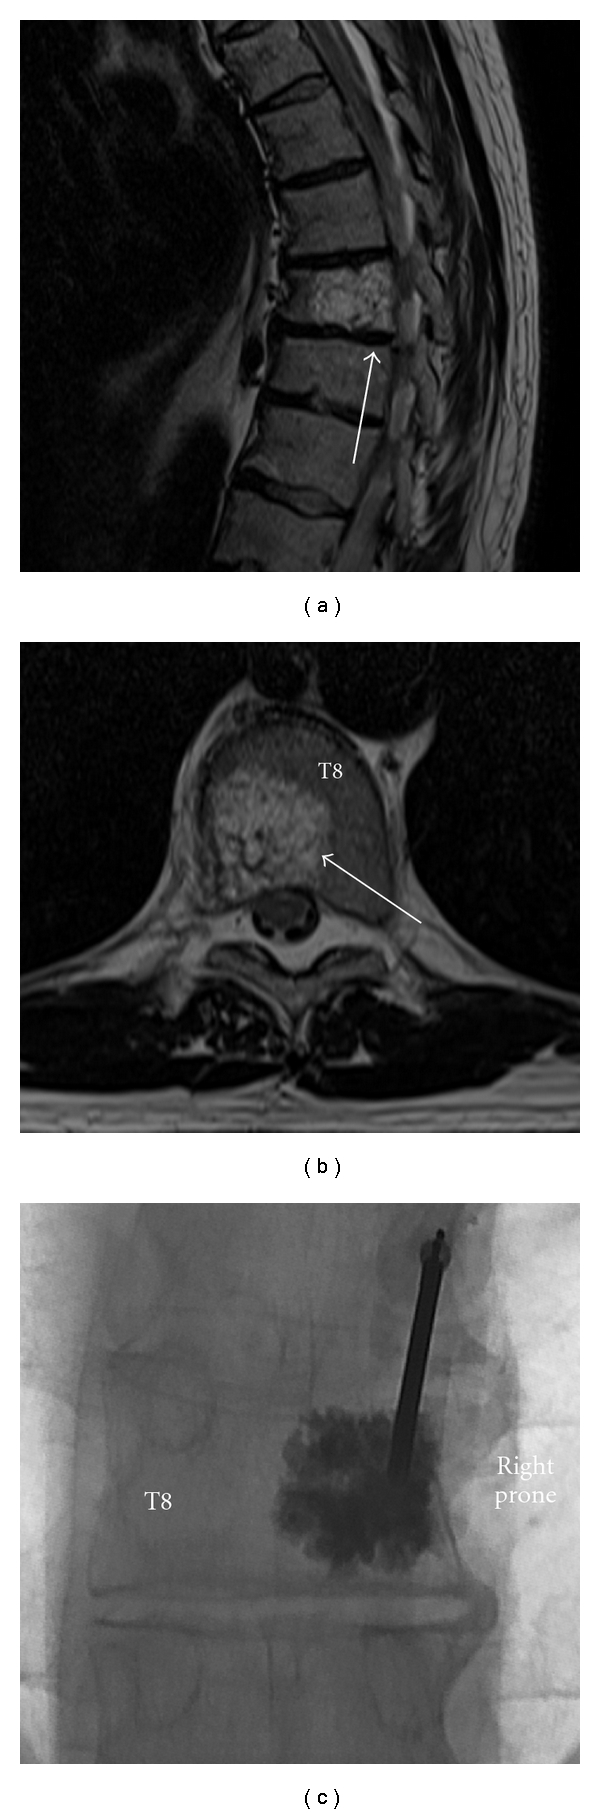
Painful haemangioma of right-hand aspect of T8 (arrowed) in a 56-year-old man treated with unipedicular vertebroplasty. Sagittal (a) and axial (b) TSE T2-weighted images. (c): Vertebroplasty spot fluoroscopy image, AP projection. Postprocedure, the patient reported complete resolution of pain.
